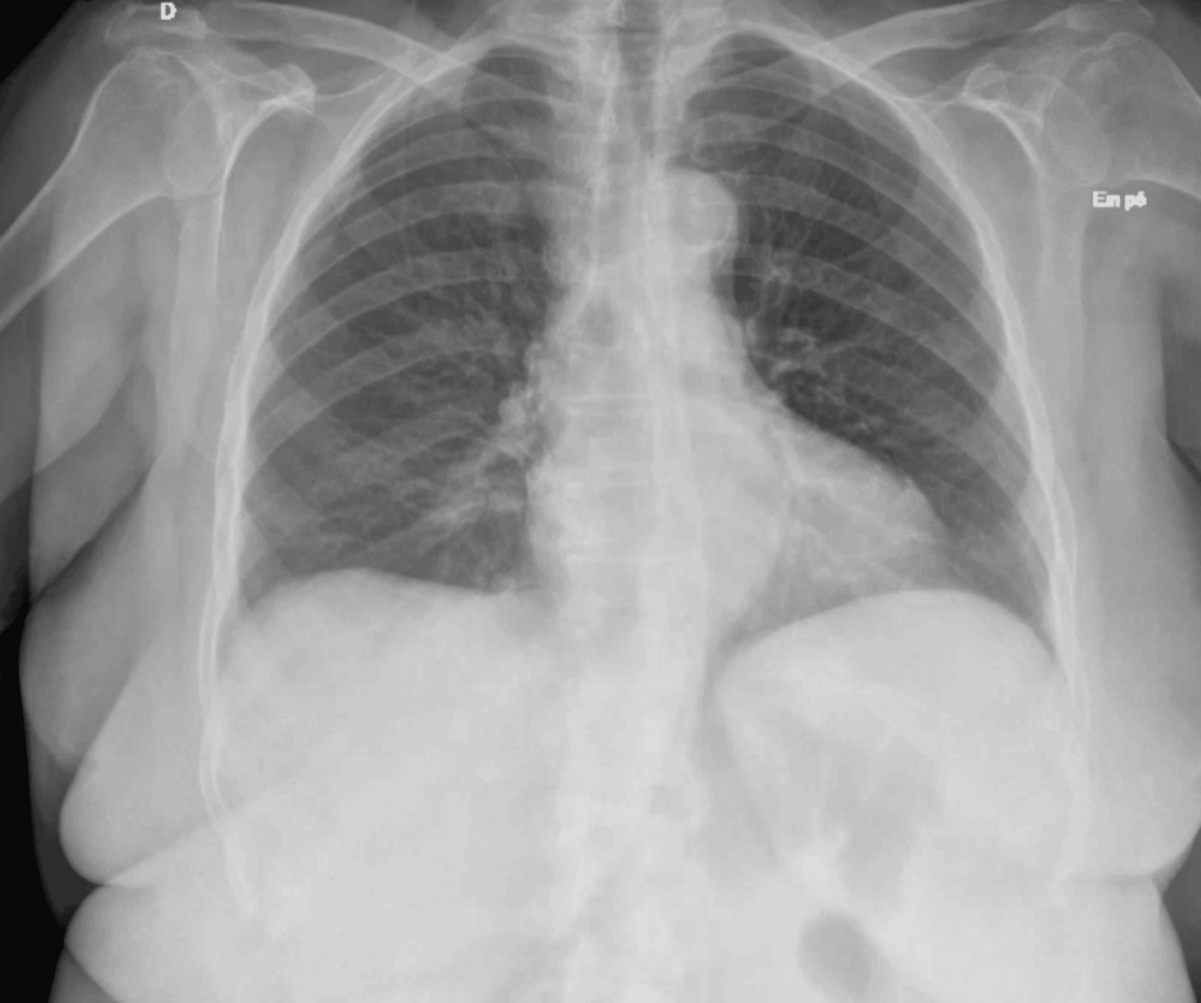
Chest radiography at admission
